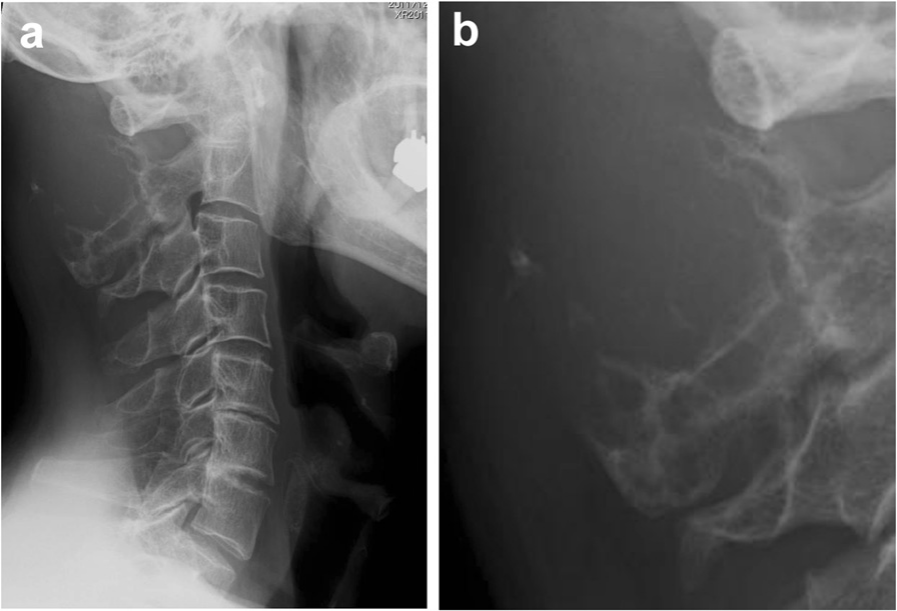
Plain radiographs of the cervical spine ((a) lateral view, (b) zoomed lateral view of the C2 spinous process) showed an expansion of the cortical contour of the C2 lamina and a soft-tissue mass with punctate calcification. Scalloping of the spinous process of C3 was also observed.
